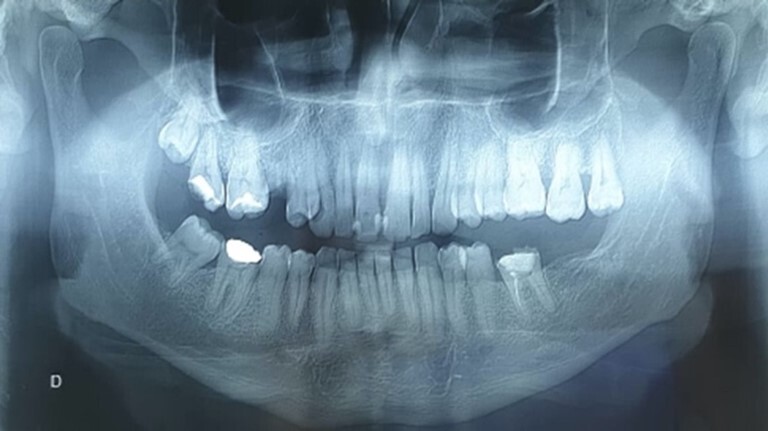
Panoramic X-ray: shows an apparent, poorly-defined anterosuperior opacity in the anterior maxilla.
